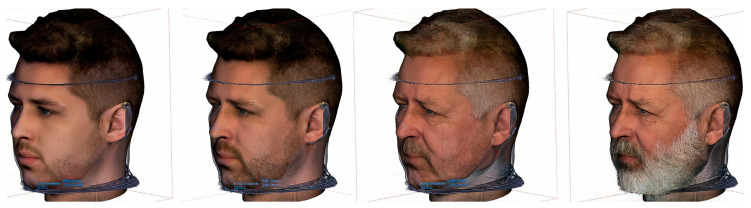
Example of CNN use of the FaceApp AI application to render the face mapped on CBCT to look younger or older. The algorithms changed just the texture and not the 3D mask, however this is probably only a matter of time. 2D face morphing based on AI or face swapping in popular videos are available and popular already a couple of years. Original face is the 2nd one.
